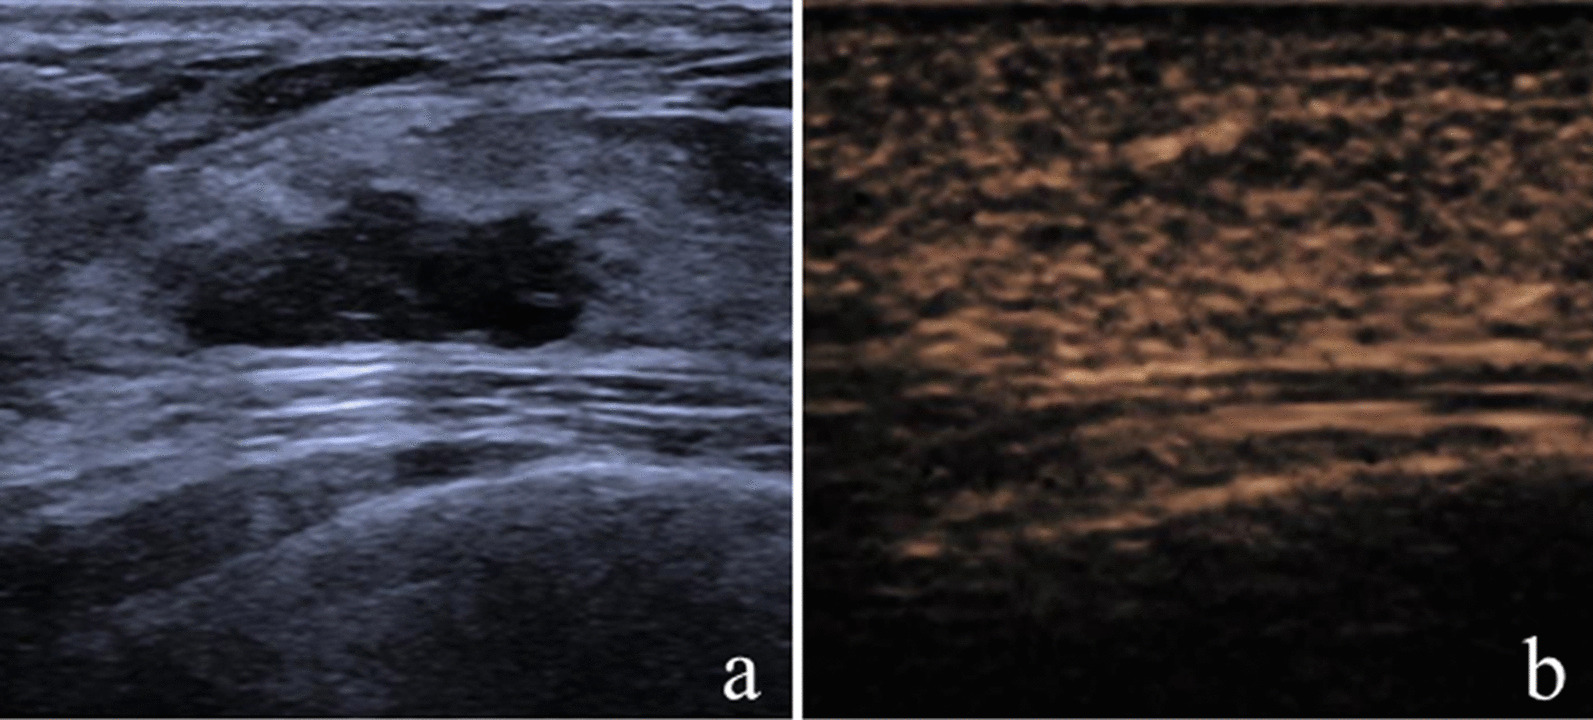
Image of a 30-year-old woman with a palpable mass. a B-mode ultrasound image; b contrast-enhanced ultrasound image showed that the lesion displayed iso-enhancement with the surrounding tissue. The CEUS score was 2 points. This lesion was originally classified as US BI-RADS 4A, downgraded to BI-RADS 3 after CEUS, and diagnosed as atypical ductal hyperplasia via US-guided core needle biopsy and as adenosis at surgery
